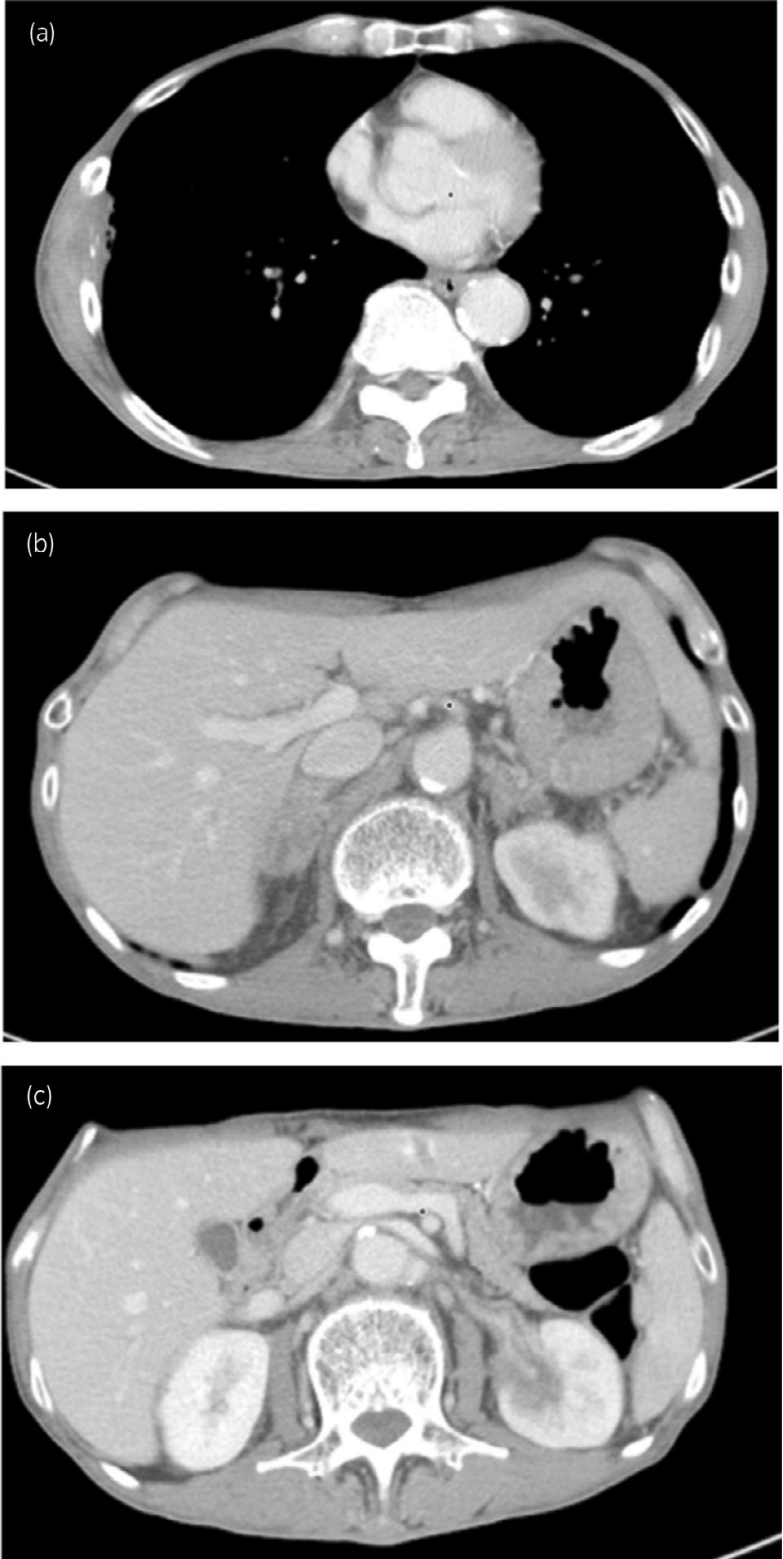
CT image 4 months after treatment. All tumor shrinkage was observed in the right thoracic wall (a), both adrenal glands (b), and the left kidney(c). We evaluated PR using RECIST 1.1.
